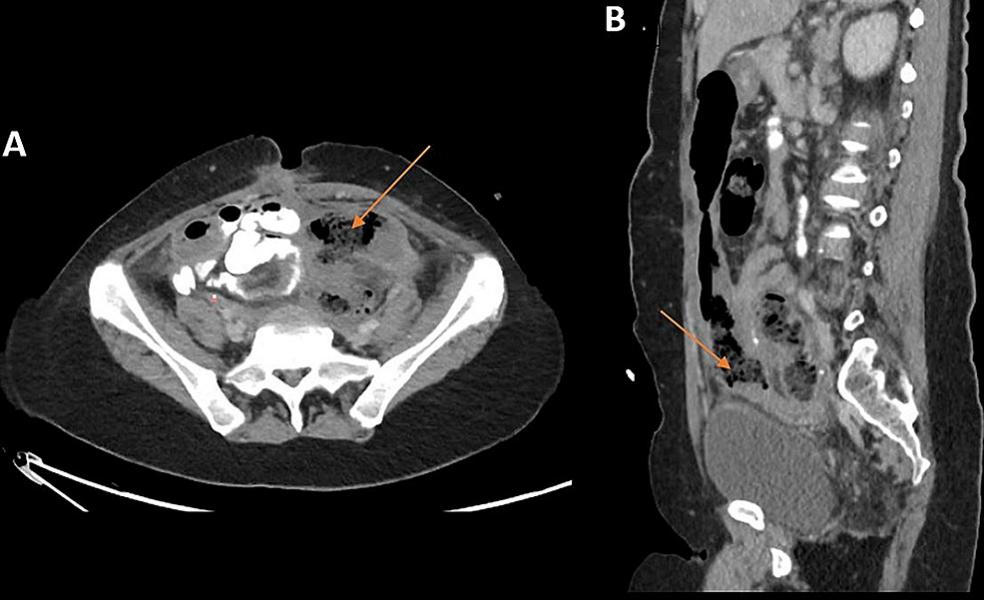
CT abdomen/pelvis with oral contrast (A-axial, B-sagittal) showing an anterior abdominal perforation with pneumoperitoneum, fluid, and feculent material (orange arrows) above the proximal anastomosis
